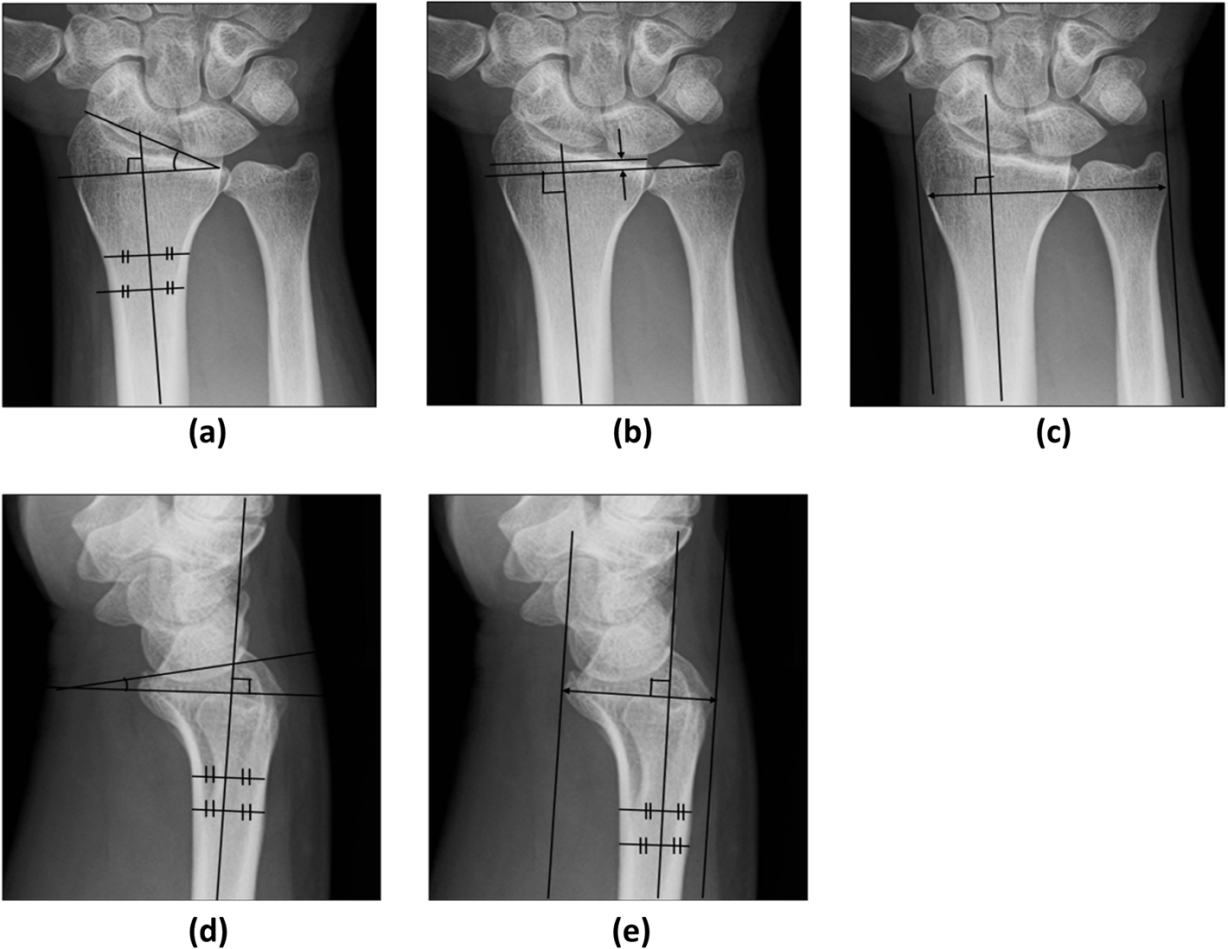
Measurements of roentgenographic indices. a Radial inclination was defined as the angle between one line connecting the radial styloid tip and the ulnar aspect of the distal radius and a second line perpendicular to the longitudinal axis of the radius. b Ulnar variance was defined as the relative lengths of the distal articular surfaces of the radius (middle line of the surface) and ulna (fovea). c The transverse diameter was defined as the distance between the radial edge of the radius and the ulnar edge of the ulna at the level of the radio-carpal joint. d Volar tilt was defined as the angle of the distal radial surface with respect to a line perpendicular shaft. e The antero-posterior diameter was defined as the distance between the volar and dorsal edge of the distal radius
